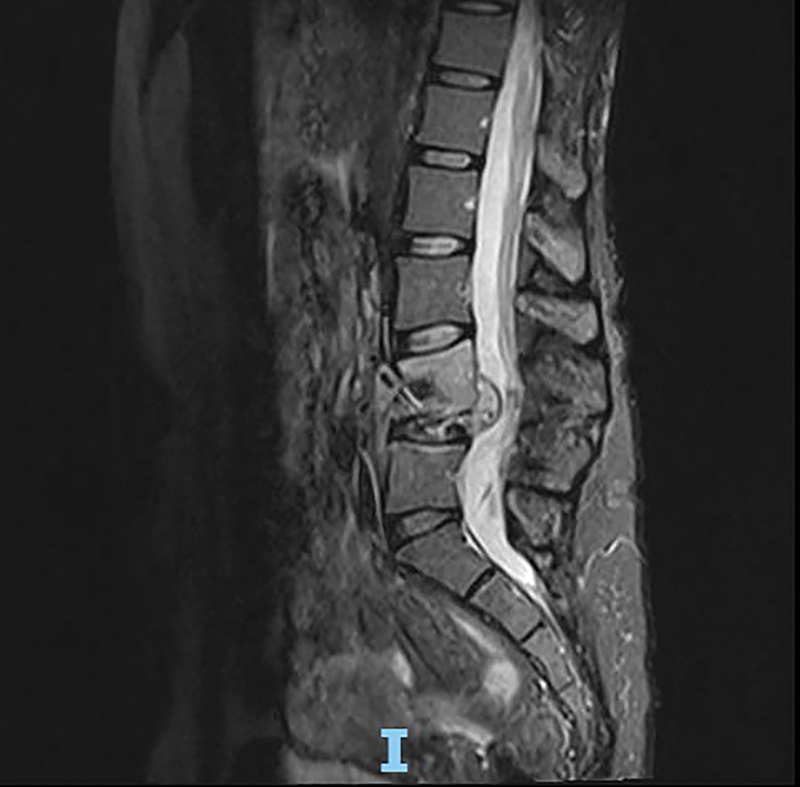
Sagittal short tau inversion recovery (STIR) magnetic resonance imaging (MRI) sequence of the lumbar spine, showing signs of total collapse of the L4 vertebral body with foraminal compression and spinal cord sclerosis in patient 1.
